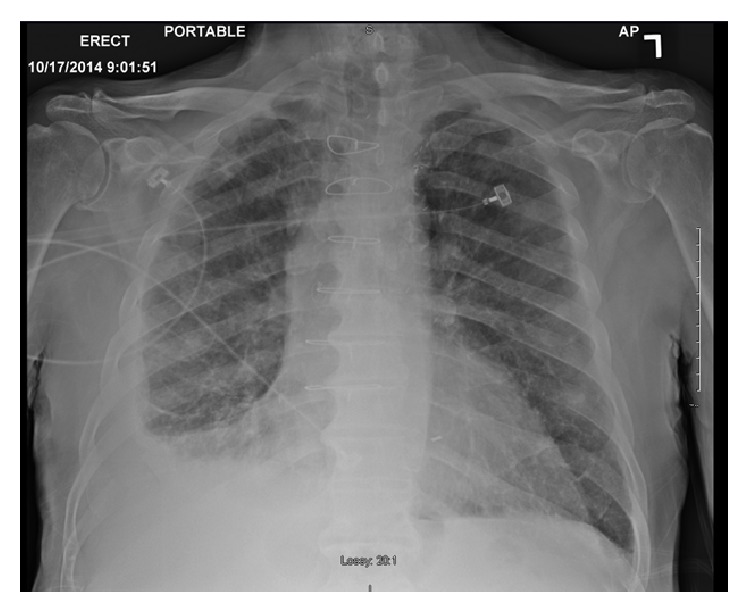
Chest X-ray showed a right lung infiltrate and moderate right pleural effusion, small left pleural effusion, bilateral small irregular opacities, and pleural thickening.
